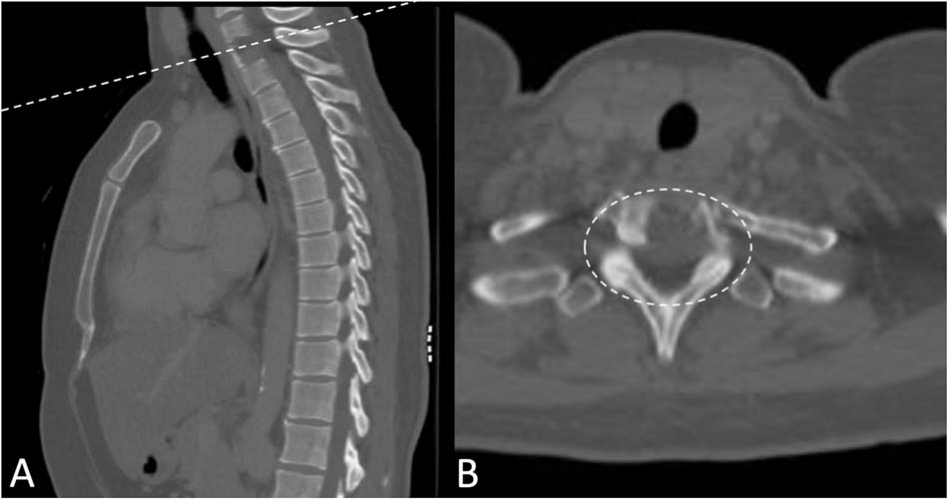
Preoperative CT Scan December 2019. Sagittal view of oligometastatic lesion at T2 vertebral body. (A) Sagittal view of oligometastatic lesion at T2 vertebral body. (B) Transverse view determined ESCC grade 2 as there is spinal cord compression, but with cerebrospinal fluid visible around the cord.
